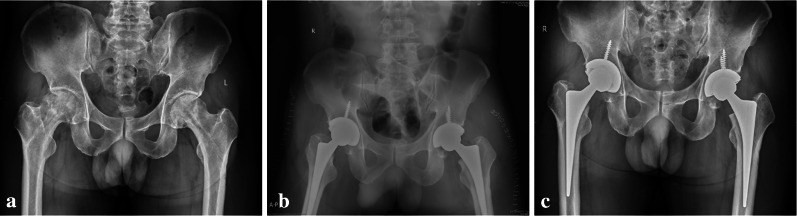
a Preoperative anteroposterior (AP) hip radiograph of 49-year-old male patient with bilateral femoral head osteonecrosis; b X‐ray images 3 days postoperatively showing hip prosthesis in a good position (right: DAA-THA; left: PLA-THA); c AP hip radiograph at 12-month follow-up showing the prosthesis in a good position without loosening
